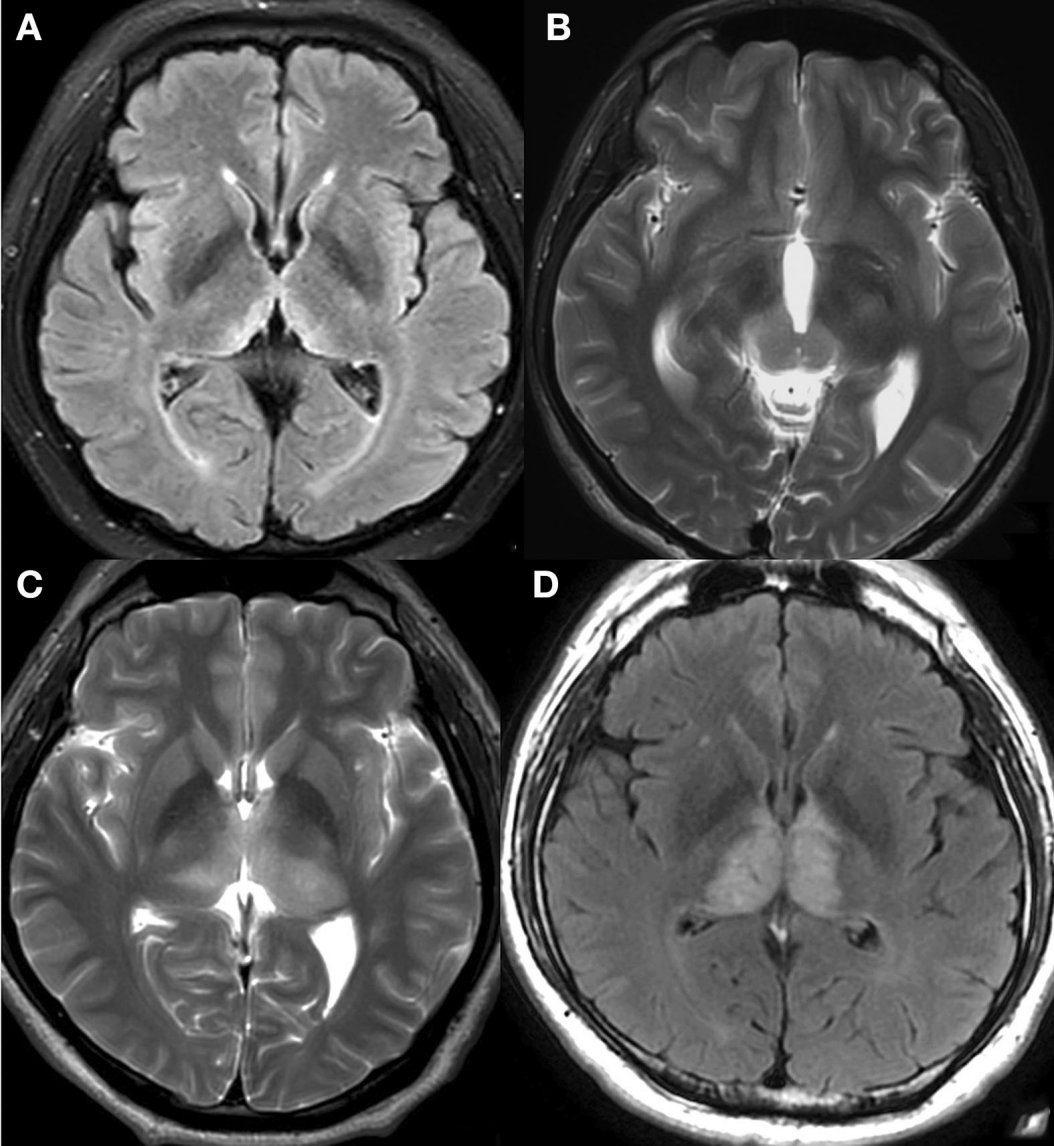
Negative and positive volume mismatch signs. (A) A 46-year-old woman with mental depression for 3 months, memory loss for more than 1 month, limb numbness and weakness for half a month. T2-FLAIR revealed hyperintensity on paramedial bithalami with negative volume mismatch. Observers predicted that the lesions might not be tumorous, and this prediction was later confirmed when the patient developed WE. (B) A 22-year-old man with no specific symptoms. T2WI revealed high signal intensity on medial parts of both the bilateral thalamus and the cerebral peduncle with positive volume mismatch. Germinoma was pathologically confirmed and the observers' prediction was correct. (C) A 43-year-old man with right limb numbness for more than 3 years, and aggravated limb numbness with diplopia for 2 months. T2WI showed heterogeneous high signal intensity on bithalamus with positive volume mismatch. Anaplastic astrocytoma (AA) was pathologically confirmed, and the observers' prediction was proven correct. (D) A 41-year-old man with aggravated memory loss for more than 2 months. T2-FLAIR revealed abnormalities of both thalami without volume mismatch sign. MRI venography showed a complete absence of flow within the straight sinus, confirming DCVT. The observers' prediction was correct.
